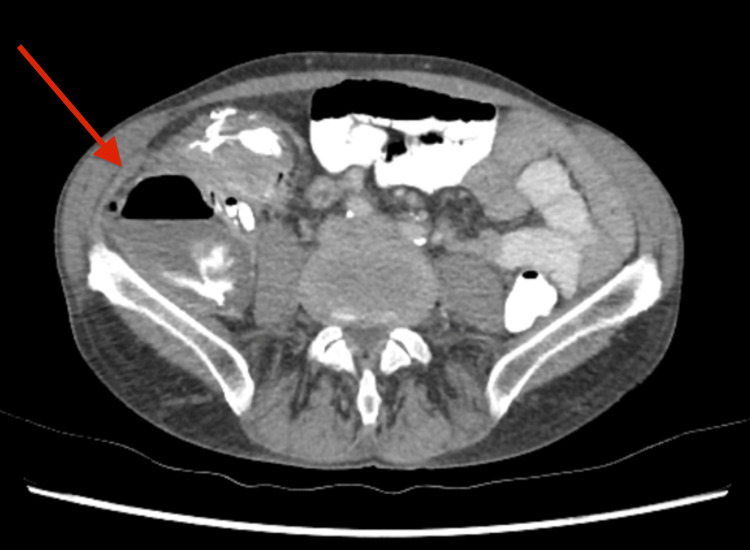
CT abdomen with oral contrast demonstrating extravasation of contrast into intra-abdominal collection
